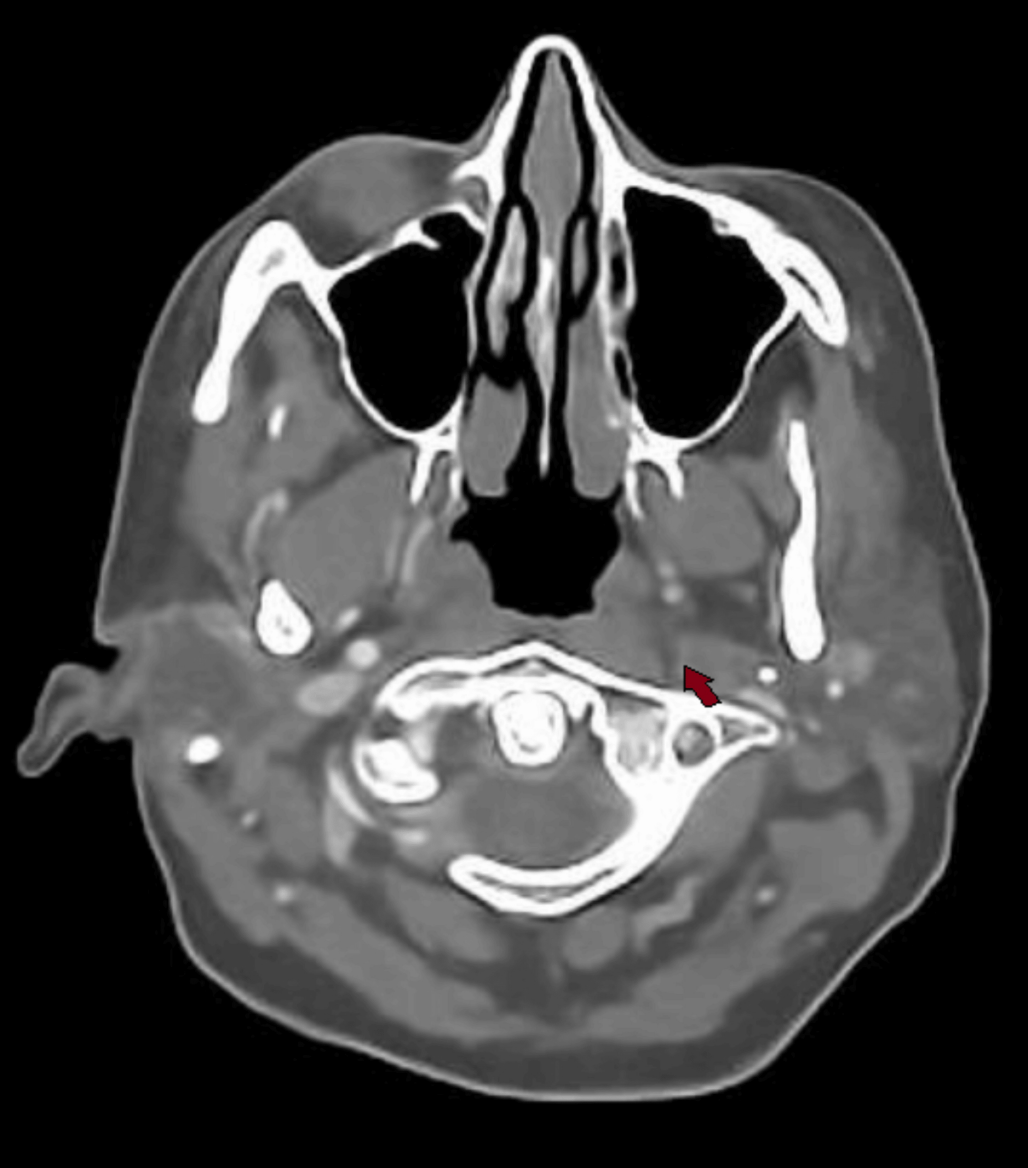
Axial CT carotid angiogram showing a lack of contrast flow in the left internal carotid artery beyond the dissection.
